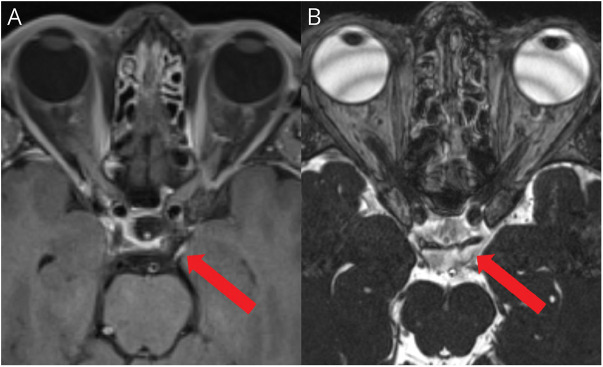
MRI Imaging Findings (A) Axial T1 postcontrast MRI demonstrating smooth enhancement of the cisternal portion of the left oculomotor nerve. (A) CSF filled dural cuff surrounds the nerve, which is susceptible to inflammatory, compressive, or neoplastic pathology.^2^ (B) The 3D constructive interference in steady state sequence is useful for visualizing abnormal signal within the nerve (red arrow).
